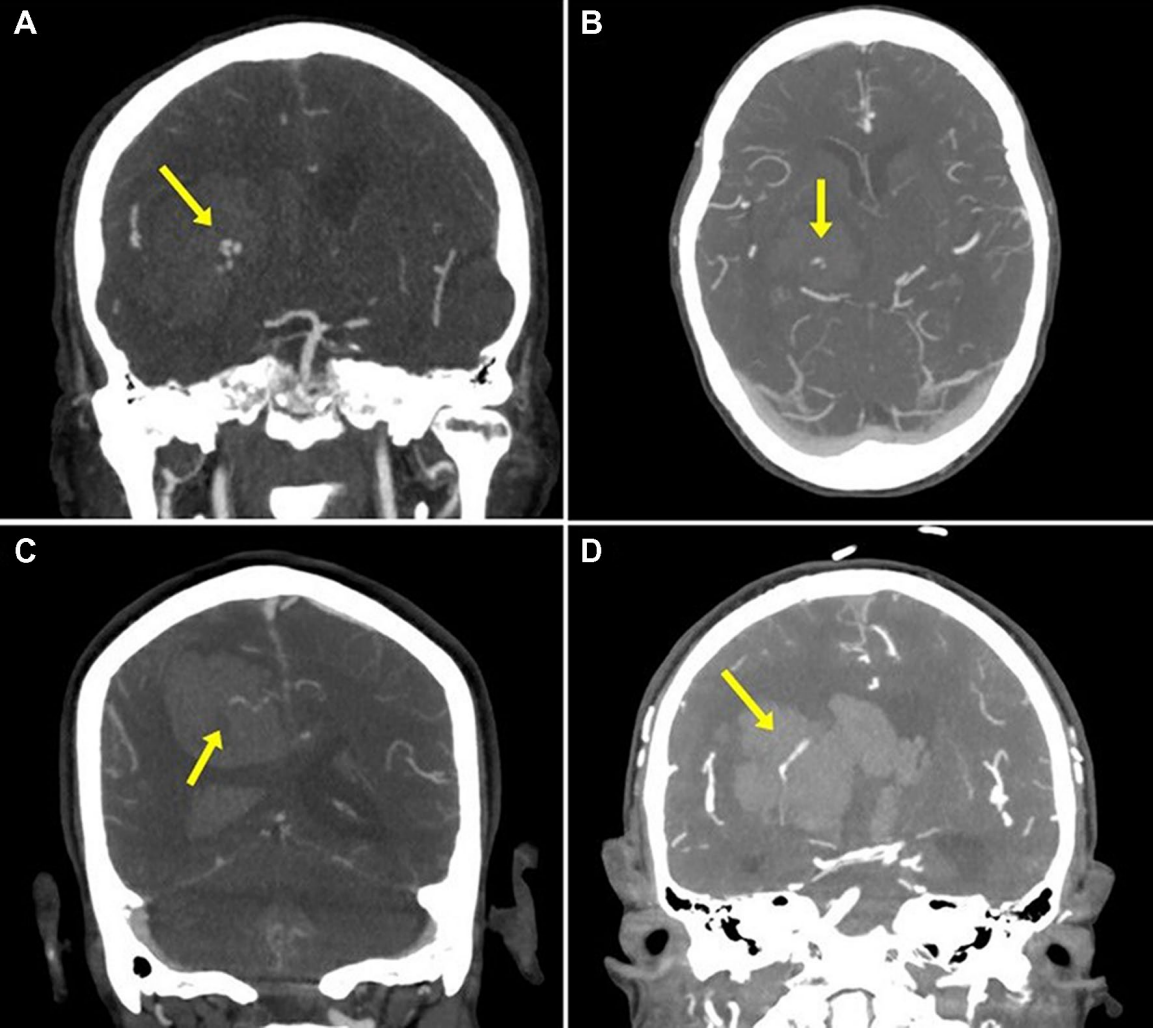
Computed tomography angiograms demonstrating examples of spot sign (yellow arrows) in four different patients. The appearance of the spot sign was variable, including examples with multiple associated foci of hemorrhage (a), small, isolated foci of hemorrhage (b), as well as streaks of extravasation appearing to come from branches of the anterior cerebral artery (c) or the branches of the middle cerebral artery, with lenticulostriate arteries particularly being a frequent apparent source of extravasation (d)
